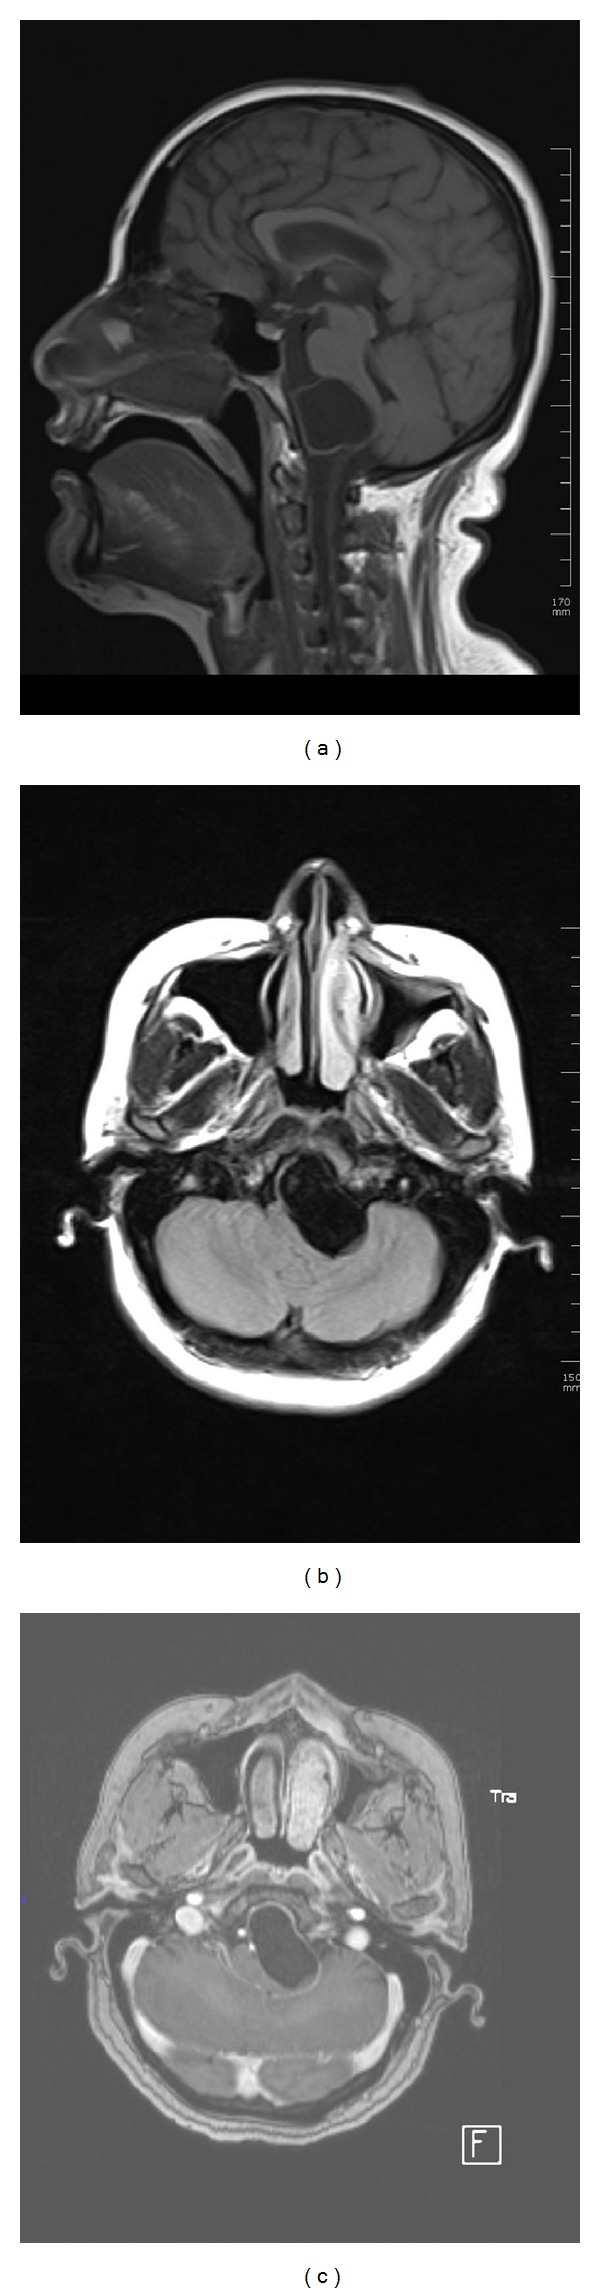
(a) T1W sagittal, (b) FLAIR transverse, and (c) postcontrast T1W transverse images. An unhanced cystic mass is seen in the premedullary space. The slightly left parasagittally located mass is compressing the brainstem left anteriorly.
